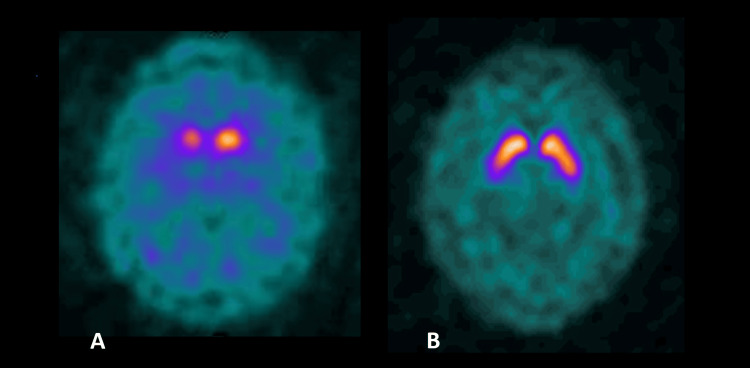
A. A 52-year-old patient’s DaTSCAN SPECT image showing significantly reduced, asymmetric uptake of 123I-ioflupane in the striatum. Lack of tracer accumulation bilaterally in the putamen and asymmetry of tracer uptake in the caudate nuclei with significantly lower activity on the right side. Abnormal increased tracer accumulation in the background. B. Normal DaTSCAN SPECT reference image showing normal uptake in the striatum and background. DaTSCAN: Dopamine transporter scan; SPECT: Single photon emission computed tomography The attached images are fully diagnostic images of this type of examination. The single photon emission computed tomography (SPECT) method is characterized by significantly lower resolution than other imaging methods, such as CT or MRI, and the scans obtained have a ‘blurred’ component. A sharper image would require digital processing, which would not be consistent with the nature of this examination.
